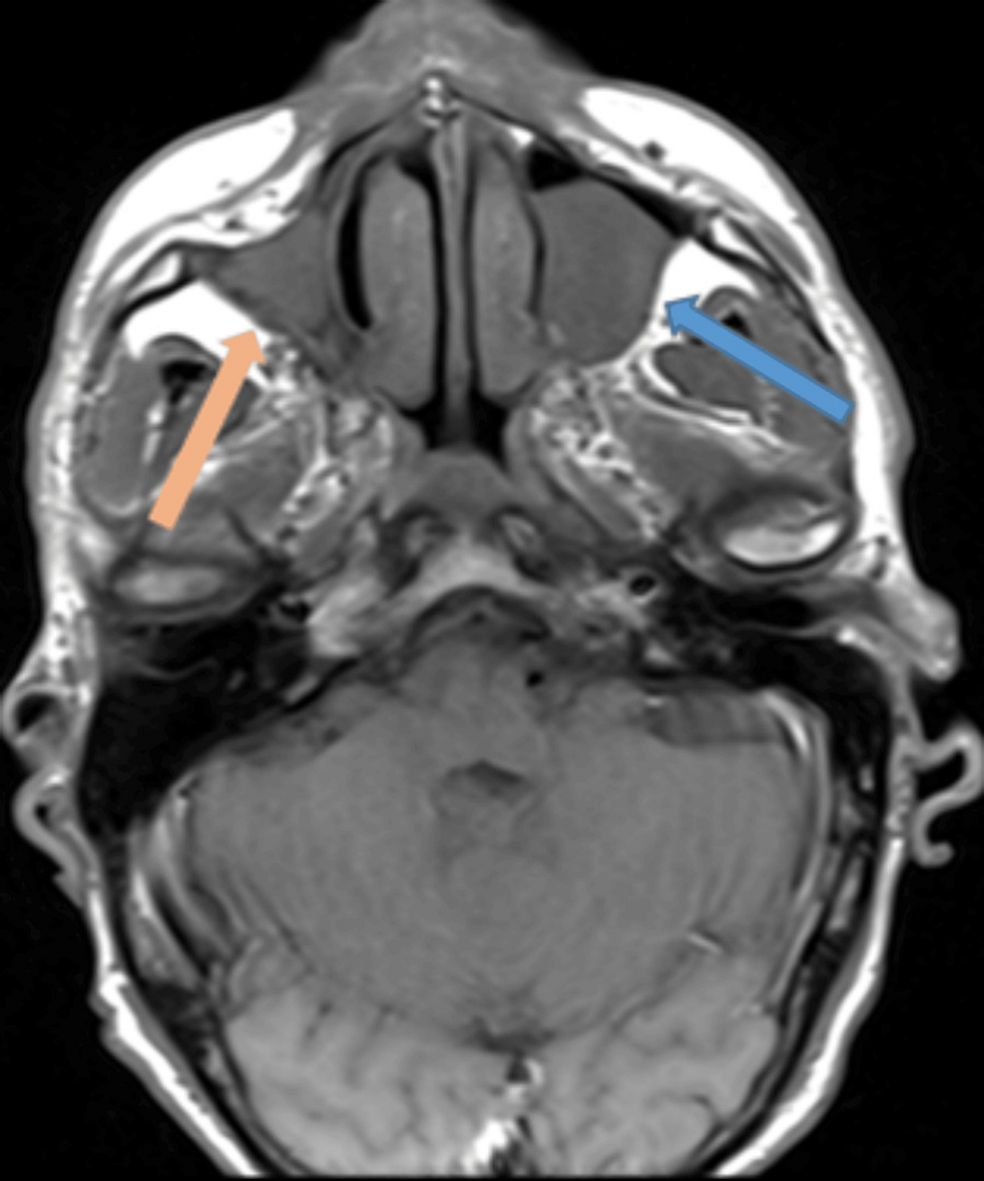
T1-weighted turbo spin echo (TSE) MRI – axial image Near complete opacification of the right maxillary with loss of the sinus cavity volume (orange arrow). There has been mild progression in the left maxillary sinus polypoidal thickening (blue arrow).
